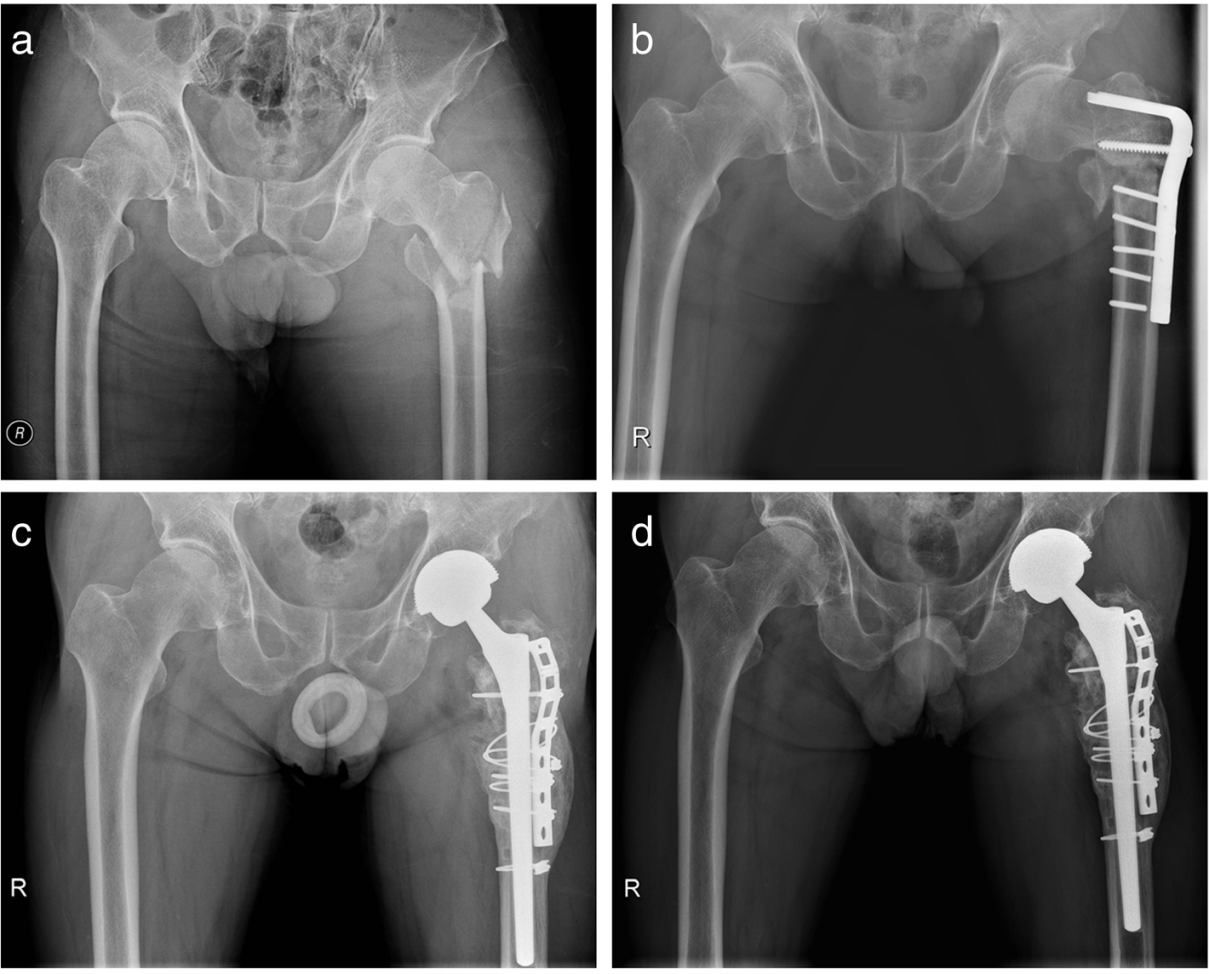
a A 69-year-old man sustained a subtrochanteric fracture of the left femur. b Preoperative radiograph showing the failure of the angled blade plate with screw breakage and nonunion of subtrochanteric fracture 15 months after internal fixation. c The plate was removed and total hip arthroplasty was performed with long femoral stem and reconstruction of fragments with the cable grip system. d Six years postoperatively, the patient returned to unassisted ambulation with a pain-free range of motion of the hip joint
